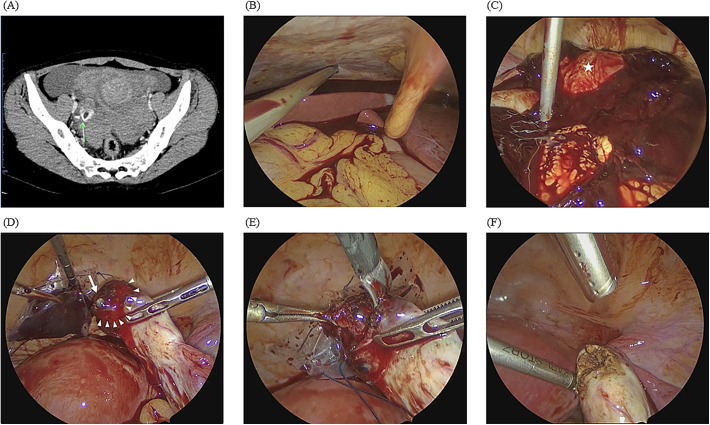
(A) Axial contrast-enhanced computed tomography scan through the pelvis showing bleeding from the right ovary (green arrow). Exploratory laparoscopic imaging reveals a huge amount of bleeding in the abdominal cavity, especially extending to the liver, and with the uterus visible within the hematoma (star). (D) Laparoscopic imaging showing the presence of the gestational sac on the surface of the ovary (arrow), with persistent bleeding from the ovary over a wide area (arrowhead). (E) Laparoscopic imaging of the wedge resection of the pregnancy tissue using forceps. (F) Laparoscopic imaging of the electrocoagulation of the surface of the excision site to prevent residual pregnancy tissue and the development of gestational trophoblast disease. (For interpretation of the references to color in this figure legend, the reader is referred to the web version of this article.)
